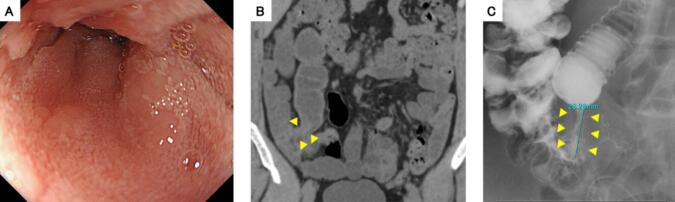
Arrowhead: stenosis of the terminal ileum. A: Lower gastrointedtinal endoscopy showed no abnormalities in the intestinal mucosa. B: Abdominal CT revealed narrowing of the terminal ileum and dilation of the oral intestinal lumen. C: Ileus tube angiography confirmed the stenosis of the terminal ileum.
